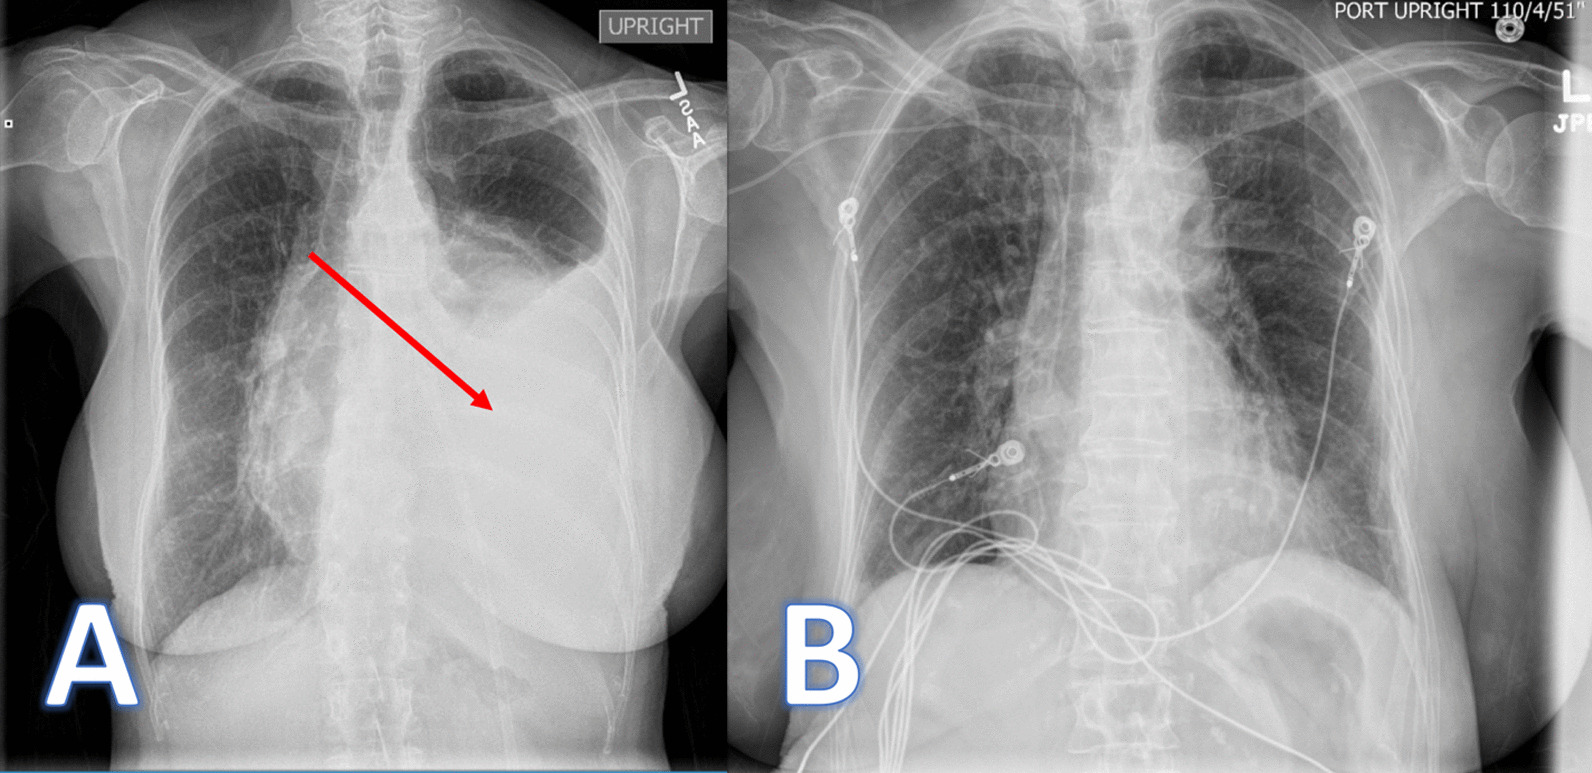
Chest X-rays. A Pre-operative chest X-ray showing SFTP. B Post-operative chest X-ray
